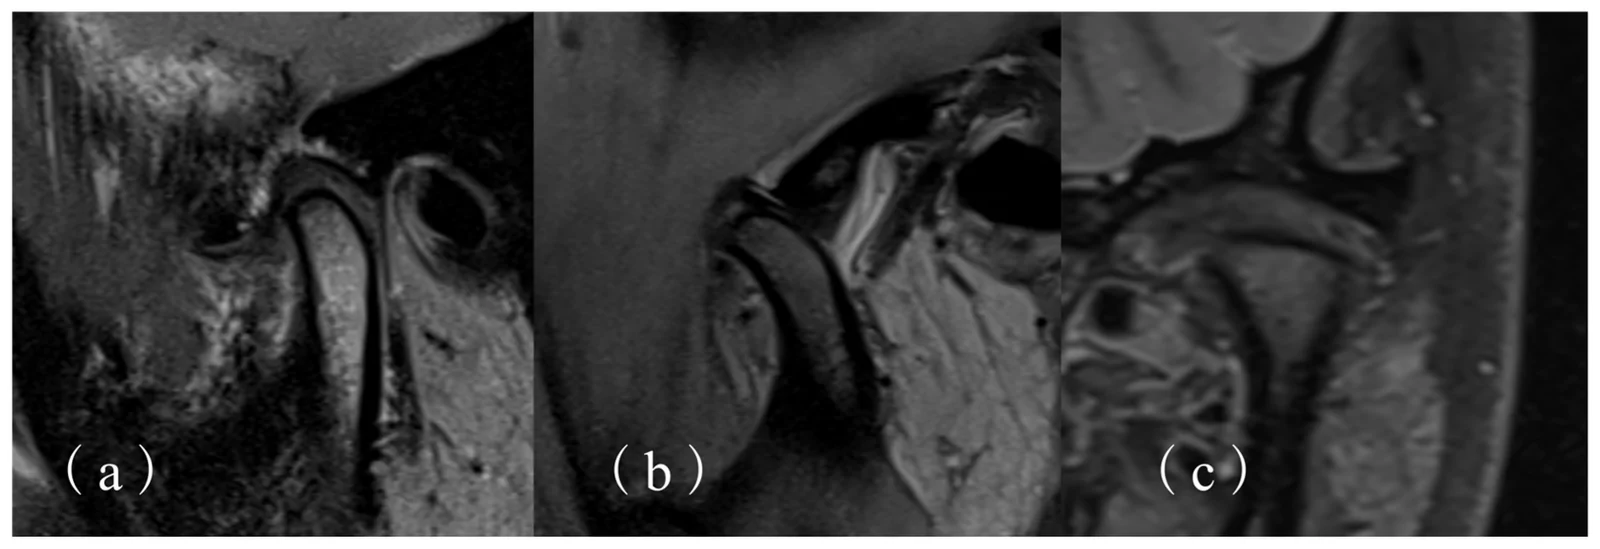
Cropped MRI images for TMJDJD classification: (a) sagittal view of a closed-mouth MRI image; (b) sagittal view of an open-mouth MRI image; (c) coronal view of an MRI image.
